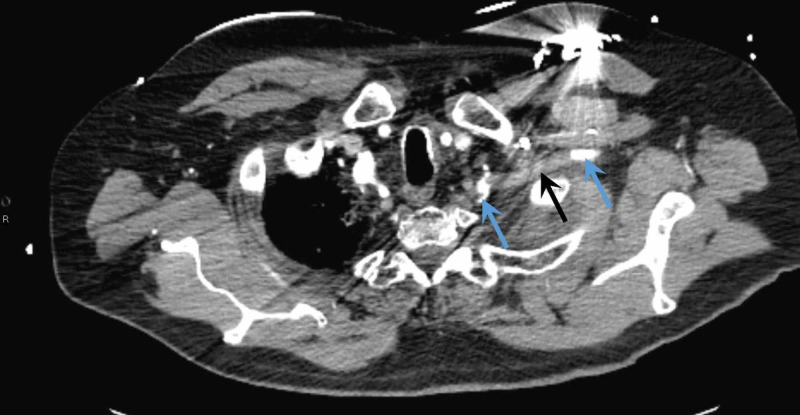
Transverse CT image with arterial phase contrast showing patent portions of the left subclavian artery (blue arrows) and the occlusion (black arrow).
